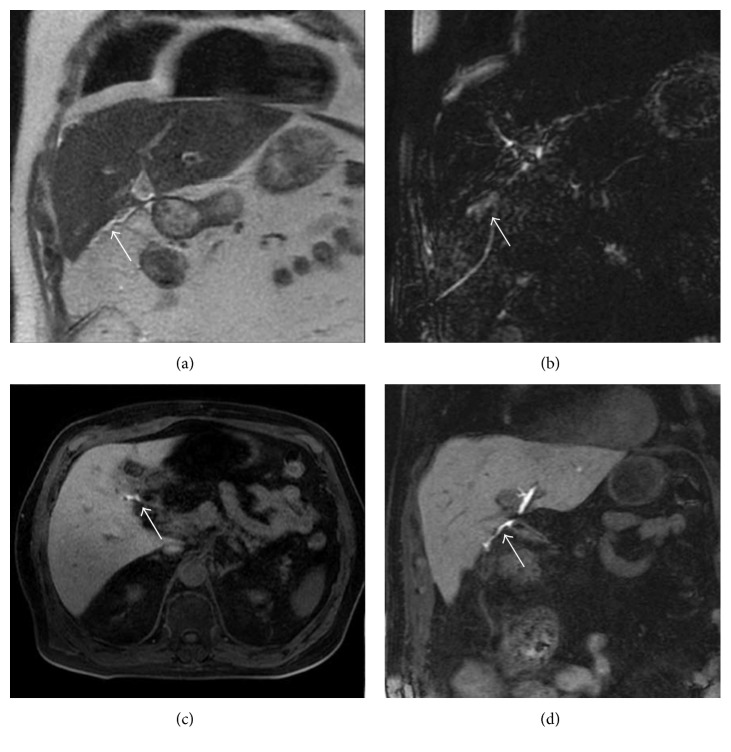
Biliary leak after cholecystectomy. Coronal T2-weighted single-shot fast spin-echo image (a) and 3D FRFSE cholangiography (b) show a small fluid collection (white arrows in (a) and (b)). Images obtained in excretory phase ((c) and (d)) show progressive opacification (white arrows): a diagnosis of biliary leak was performed.
